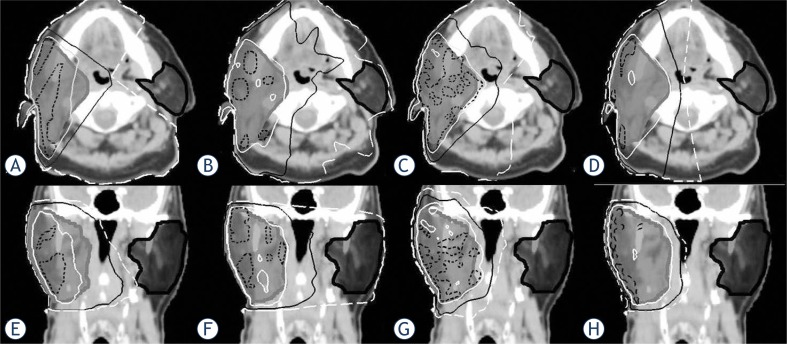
Transverse and coronal view of computed tomography images with isodose distributions at the isocenter level in a representative patient with left sided disease. (A, E) wedge pair; (B, F) 7-field IMRT; (C, G) 4-field IMRToff-axis; (D,H) 4-field IMRT co-planar. The isodoses are 105% (dashed black), 100% (white), 50% (black) and 25% (dashed white) of the prescribed dose (70Gy).
